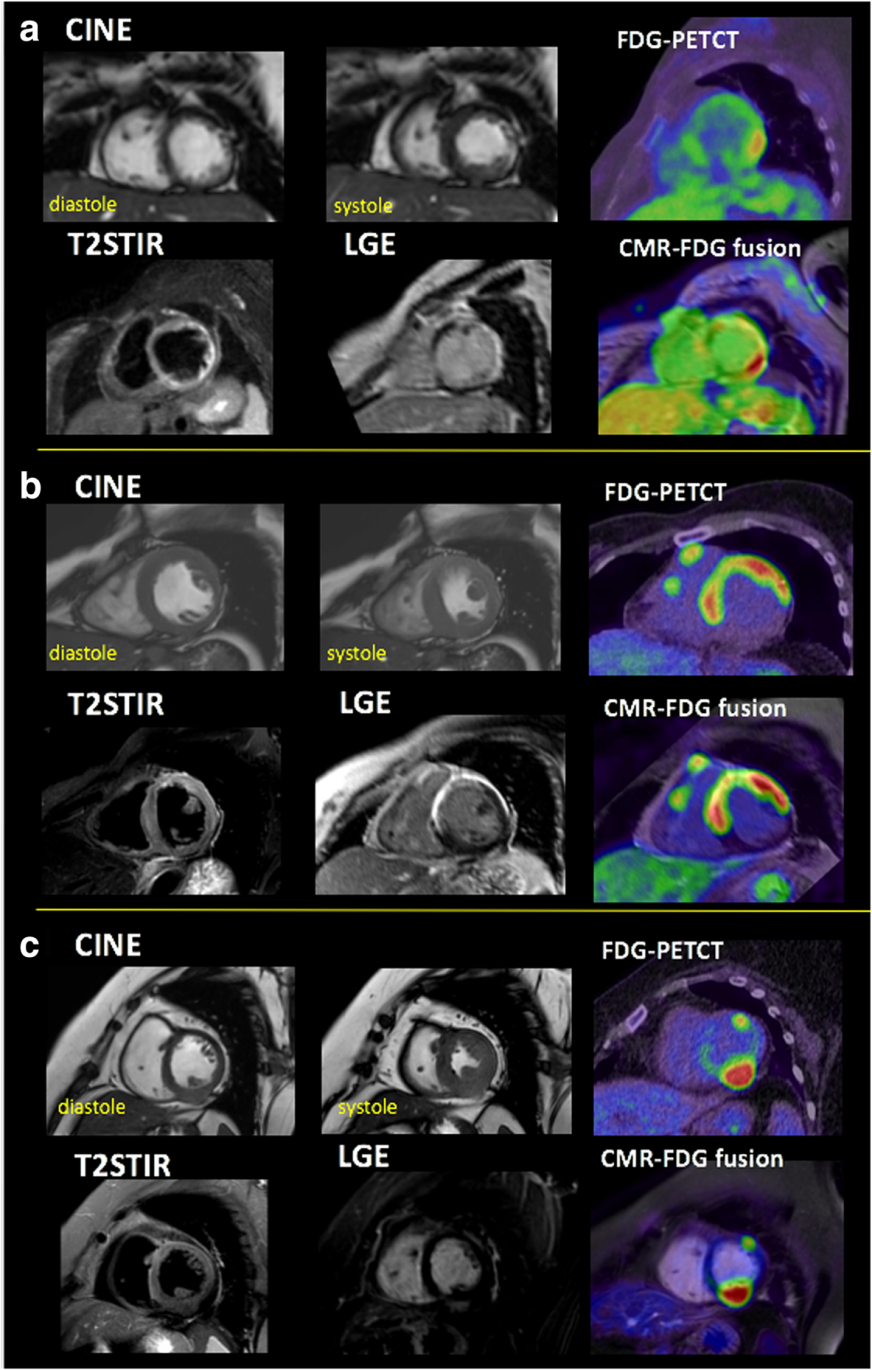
Three representative cases of CMR, FDG, and image fusion are shown. a 60-year-old female who had advanced heart failure with reduced cardiac function (EF = 30%) showed extensive LGE and dyskinetic-thinned wall in lateral. LGE volume, CMV, mismatch volume, and CMA were 45 mL, 10 mL, − 35.7 mL, and 32. FDG showed significant uptake in the area of positive LGE, and SUVmax value highest in the posterior edge of LGE site (segmental SUVmax = 6.2, LGE%wall = 54), while lower value of SUVmax = 3.4 was observed in dyskinetic lateral wall (LGE%wall = 96). b 54-year-old female with impaired cardiac function with sustained VT. CMR showed extensive LGE around basal area and significant FDG uptake (SUVmax = 9.6). LGE volume, CMV, mismatch volume, and CMA were 28.9 mL, 90.6 mL, 62 mL, and 345. Under fusion-guided analysis, basal antero-septal segment with high-grade fibrosis showed significant uptake (SUVmax 6.5). However, higher uptake (SUVmax = 9.5) was observed in basal septum and anterolateral wall which showed LGE%wall = 59. T2-weighted image showed high intensity in the antero-basal wall. c 66-year-old female who had systemic sarcoidosis. Her ejection fraction was slightly lower (LVEF = 47%). She was pointed out with premature ventricular contraction and driven for CMR. Short axis cine showed almost normal wall motion and volume in LV. However, posterior wall was remarkably thickened and with mild hyper-enhancement in LGE image (LGE%wall = 10%). In CMR-FDG fusion, significant focal FDG uptake (SUVmax = 16.6) was observed. T2 weighted image showed no abnormality. LGE volume was remarkably smaller than CMV (LGE volume, CMV, mismatch volume, and CMA were 14.6 mL, 155.4 mL, 170 mL, and 639)
